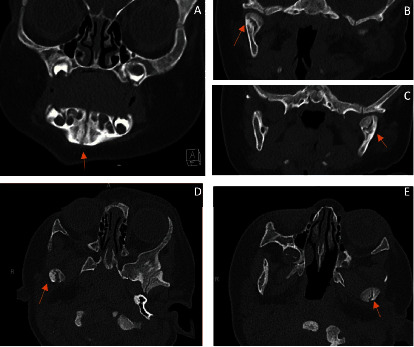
Follow-up CT scan 2 weeks after in coronal view, with no evidence of symphyseal or parasymphyseal fracture, mandibular symphysis visualized (A). Coronal view of right condylar fracture (B). Coronal view of left condylar fracture (C). Axial view of right condylar fracture (D). Axial view of left condylar fracture (E).
